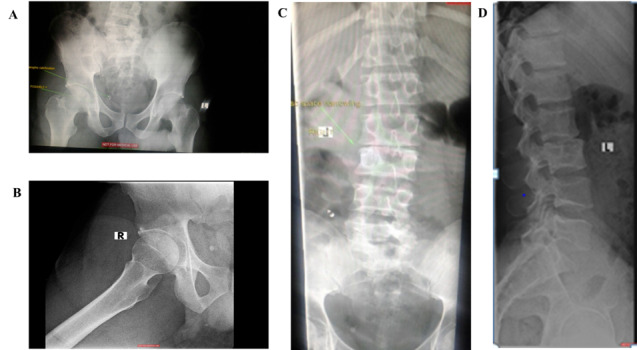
(A) X-ray of the pelvis AP view; (B) X-ray of the right hip AP view; (C) Lumbar spine X-ray, AP view; (D) Lumbar spine X-ray lateral view
